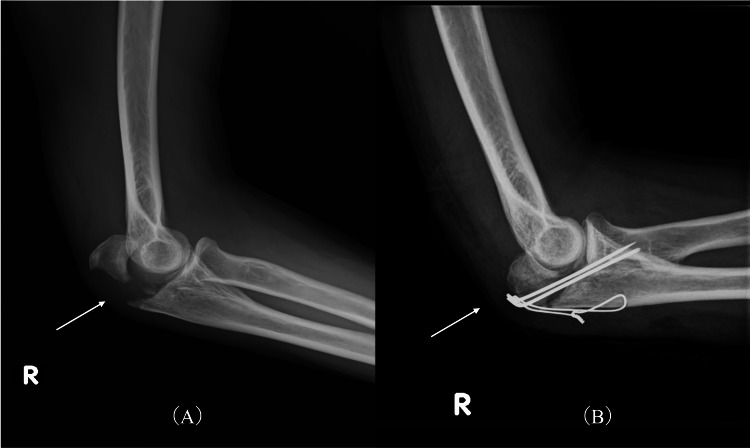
Preoperative and postoperative lateral radiographs of the right elbow (A) Preoperative lateral radiograph showing an olecranon fracture. (B) Postoperative lateral radiograph after open reduction and internal fixation with tension band wiring, showing improved alignment. The arrow in panel A indicates the olecranon fracture line. The arrows in panel B indicate the Kirschner wires and the tension band of the tension band wiring construct used for open reduction and internal fixation. The postoperative radiograph confirms anatomical reduction of the fracture and stable fixation.
